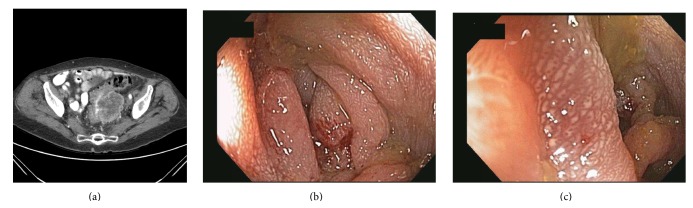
(a) CT scan revealing a 6 cm pelvic mass. (b) Colonoscopy revealing a 5 cm long stenosis in the sigmoid colon starting at 15 cm from the anal verge. (c) Colonoscopy with closer view of stenosis, which was not ulcerated but had a bluish/purplish hue to it.
